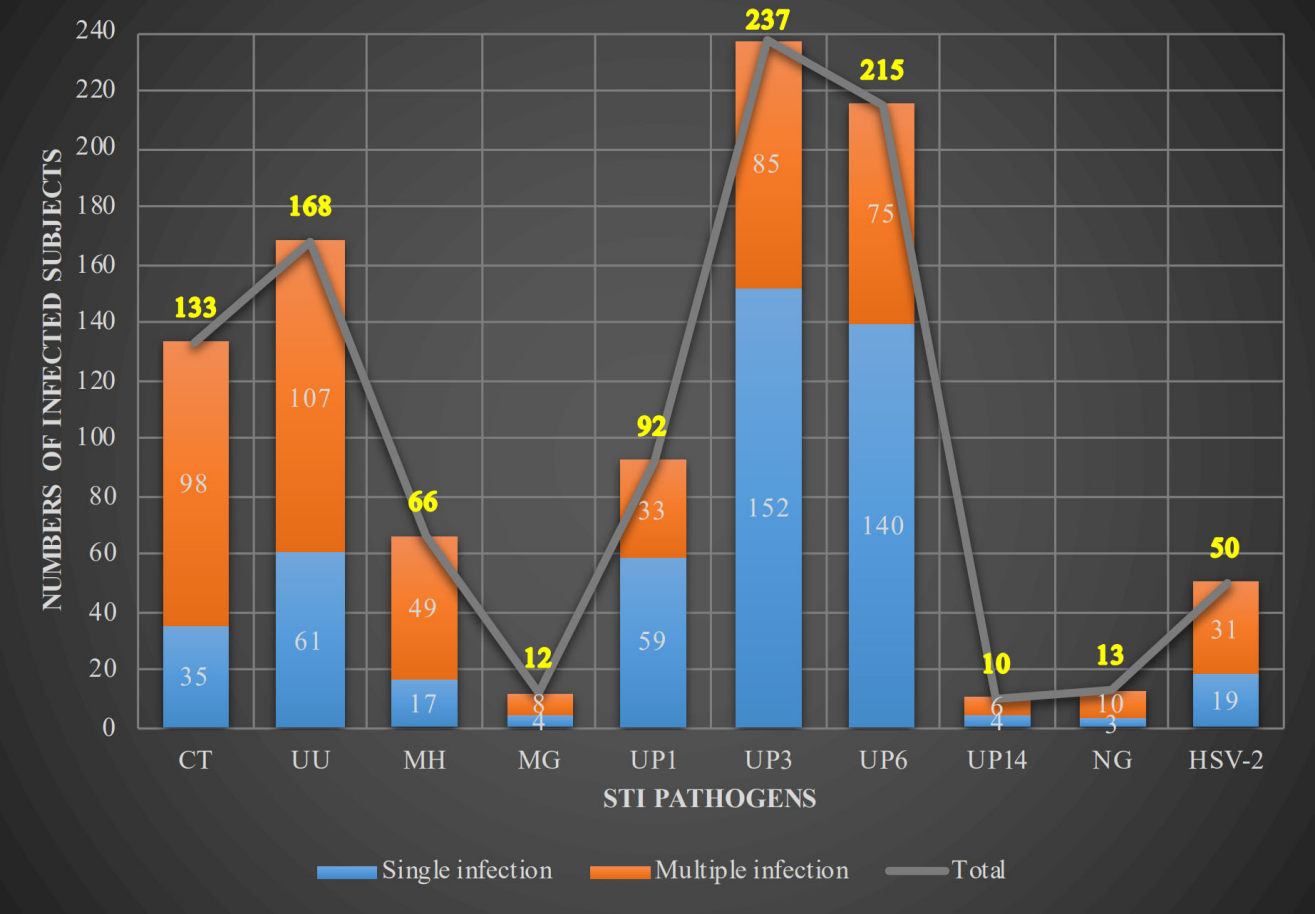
Types and proportions of the detected STI pathogens. Numbers of subjects with single infection, multiple infection, and total infection with detected STI pathogens shown. STI, sexually transmitted infection; CT, *Chlamydia trachomatis*; UU, *Ureaplasma urealyticum*; MH, *Mycoplasma hominis*; MG, *Mycoplasma genitalium*; UP, *Ureaplasma parvum*; NG, *Neisseria gonorrhoeae*; HSV-2, herpes simplex virus type 2.
